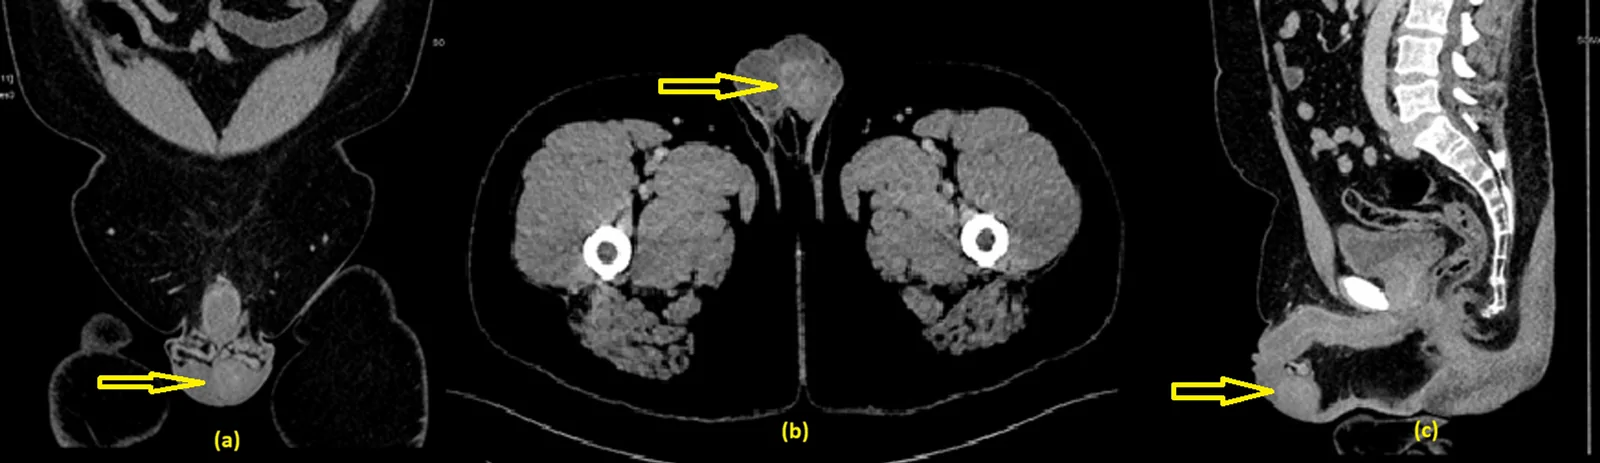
Contrast-enhanced computed tomography coronal (a), axial (b) and sagittal (c) reconstructions of abdomen and pelvis reveals a left-sided, oval, well-circumscribed, mildly enhancing extra-testicular mass (yellow arrow).
